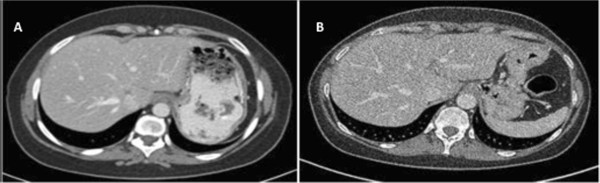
Tumor response after NKTR-102 discontinuation. A: Partial response at the 12th cycle of pegylated irinotecan NKTR-102. B: Abdominal computed tomography scan four months after the last course of pegylated irinotecan NKTR-102, showing additional response.
